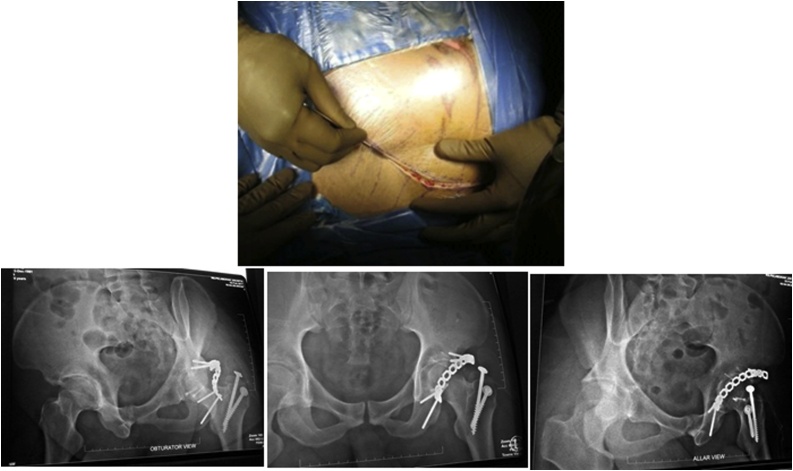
Internal Fixation through Kocher Langenback Approach & Postoperative X-ray After Surgery.
